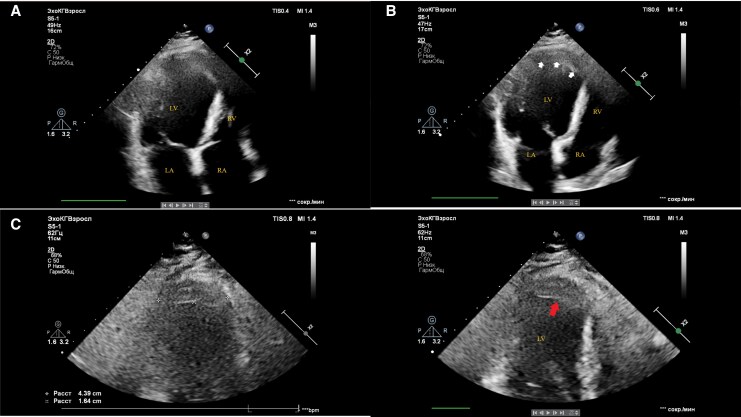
Transthoracic echocardiography findings. *(A)* Apical four-chamber view showing overall cardiac anatomy. *(B)* Apical four-chamber view demonstrating a parietal apical thrombus (white arrow) and apical-septal aneurysm. *(C)* Apical two-chamber view showing the dimensions of the apical thrombus (dotted crosses). *(D)* Apical two-chamber view highlighting the apical thrombus (red arrow) and left ventricular apical-septal aneurysm. These images demonstrate marked left ventricular dilation, apical akinesia, reduced contractility, and a left ventricular ejection fraction of 35%, consistent with severe systolic dysfunction.
